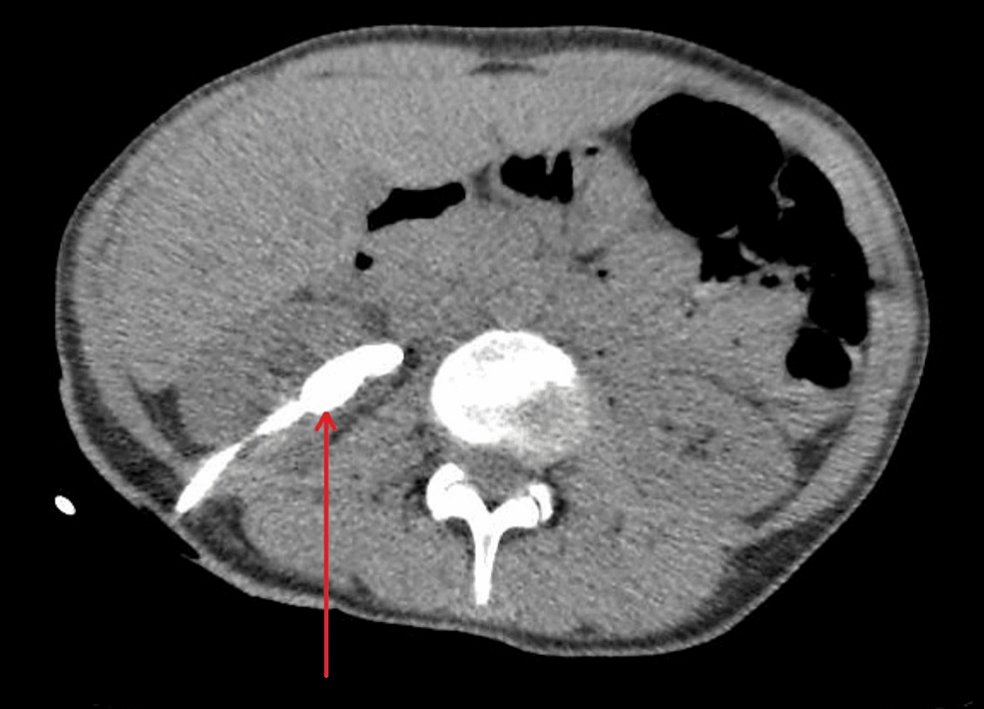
Non-contrast CT scan of the kidneys demonstrating an encrusted coil of the nephrostomy tube within the right kidney (red arrow).
